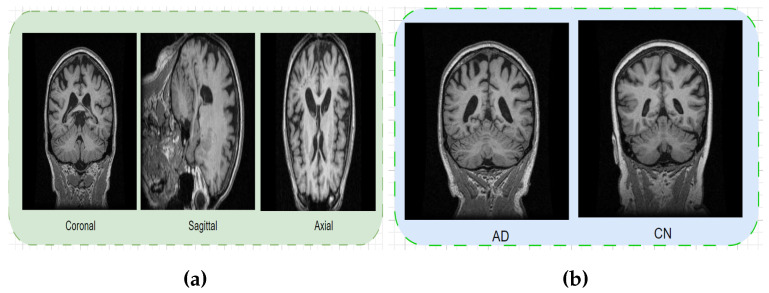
Visual information of brain MRIs: (a) Different view of MRI imaging plans, (b) Normal brains and affected by AD.
